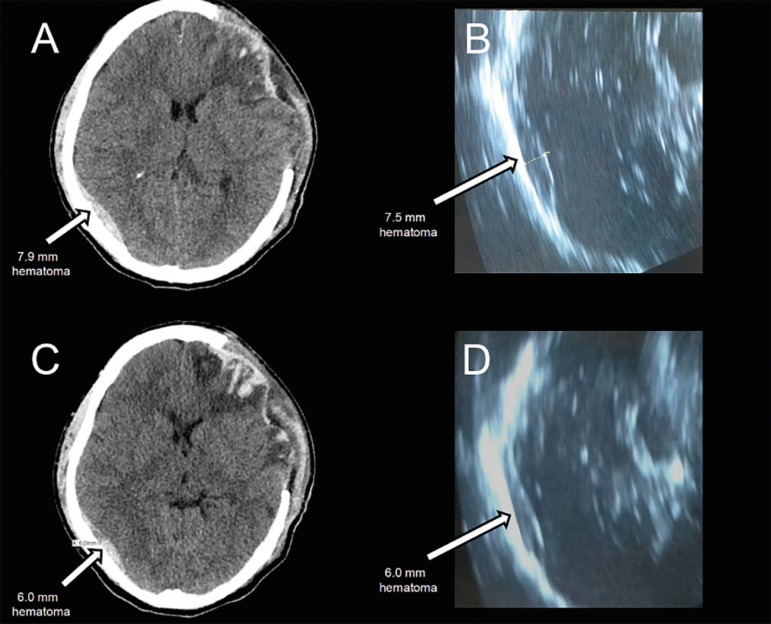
Cranial computerized tomography ultrasound scans following surgery. (A) Day 2
